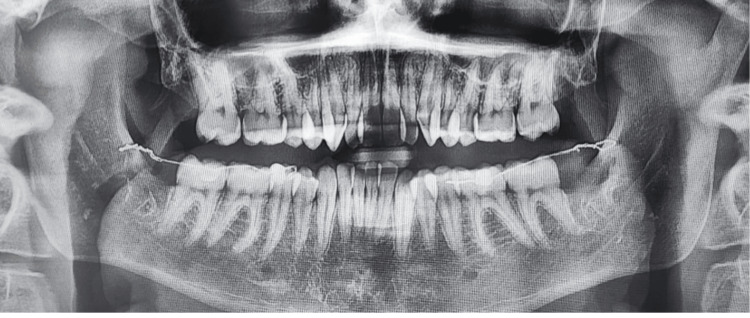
Postoperative panoramic radiograph confirming correct positioning of the bilateral Abalakov anchorage system.
